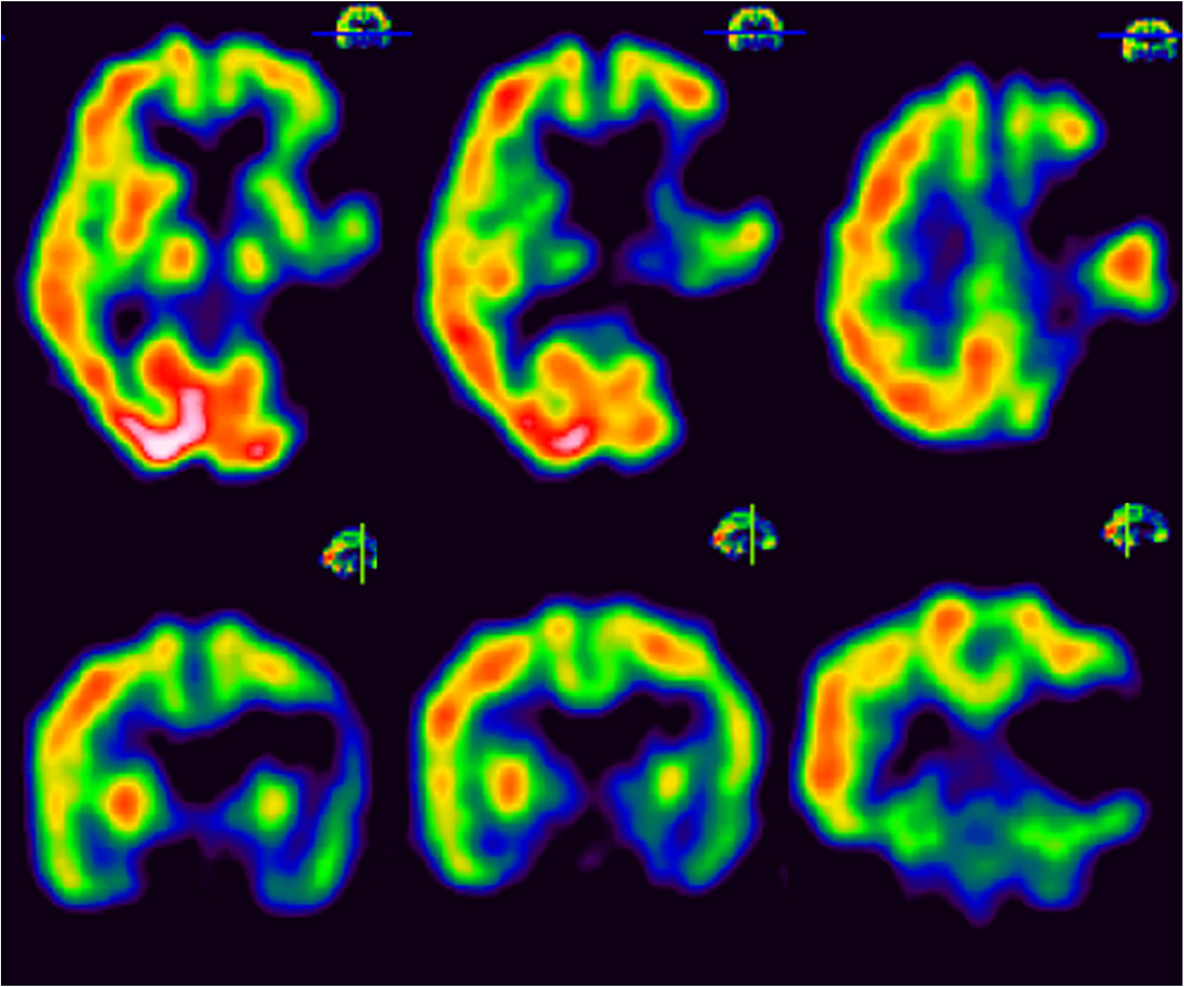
Brain single photon emission computed tomography (SPECT) image at 14 months after stroke onset. No radioactivity was seen in the left frontal and left parietotemporal regions. However, a relatively high level of radioactivity uptake was noted in the right thalamus, frontal and occipital regions
